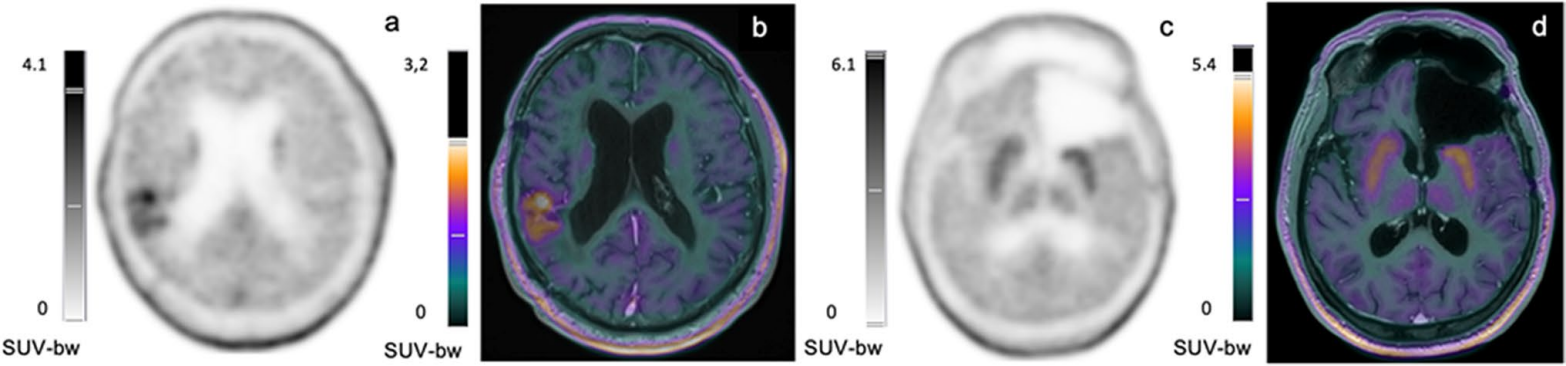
(a)(c) Static [18F]FDOPA PET images; (b)(d) Co-registered PET and MRI images (fused). The two left panels are those of an 81-years-old man with an IDH-wildtype glioblastoma with respective PET parameters obtained after 22 TMZ cycles: TBR_peak_: 2.3, MTV: 7.3 mL. Follow-up revealed progression at 3.9 months. The two right panels are those of a 54-years-old man with an IDH-wildtype glioblastoma with respective PET parameters obtained after 6 TMZ cycles: TBR_peak_: 1.5, MTV: 0 ml. Follow-up revealed progression at 12.5 months
